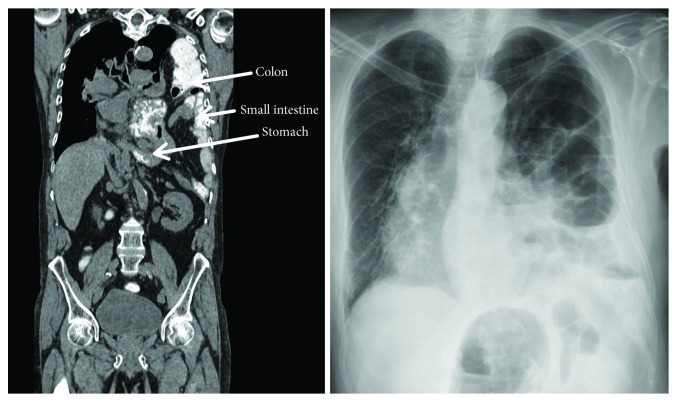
Coronary CT scan and chest X-ray 8 days after admission with large diaphragmatic hernia.
